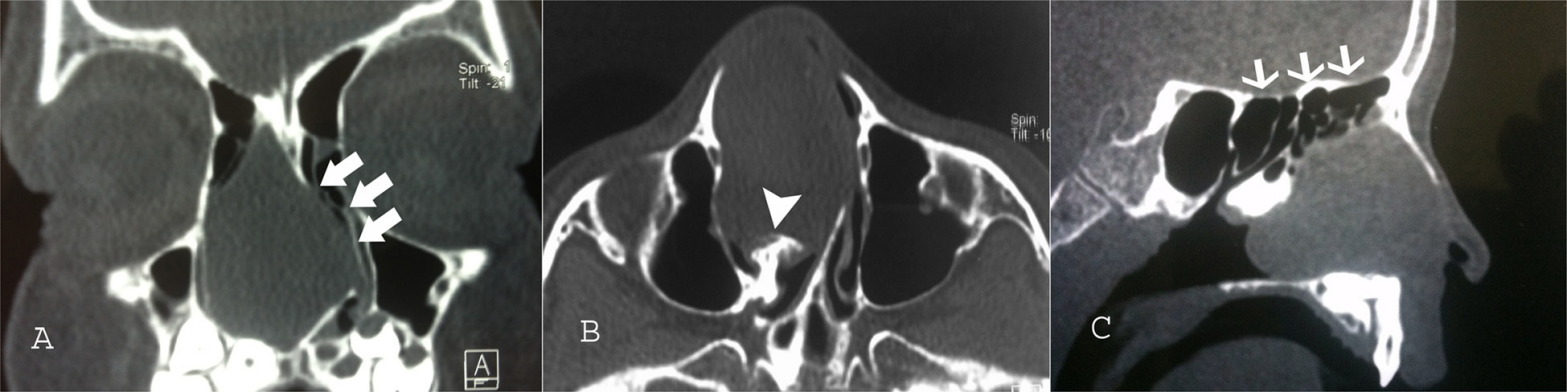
Coronal (A), axial (B), and sagittal (B,C) paranasal sinus computed tomography images showing a mucocele of the nasal cavity. (A) Coronal image showing the extensive mucocele filling the nasal cavity without orbital extension. The nasal septum has deviated to the left side due to the mass effect (white arrows). (B) Axial image showing the originating site of the mucocele from the middle concha (white arrowhead). (C) Sagittal image of the mucocele. Note that the cranial base is intact without extension into the cranial fossa (thin white arrows).
